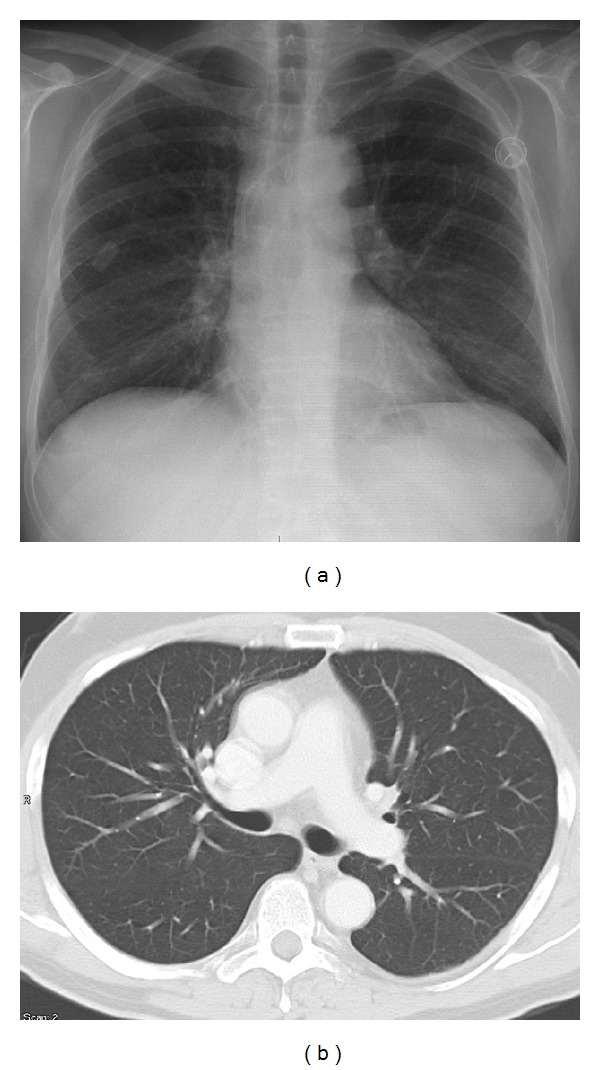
X-ray (one day after inpatient admittance) and CT scan of the chest (one month prior to first chemotherapy administration). Normal finding in both lungs.
